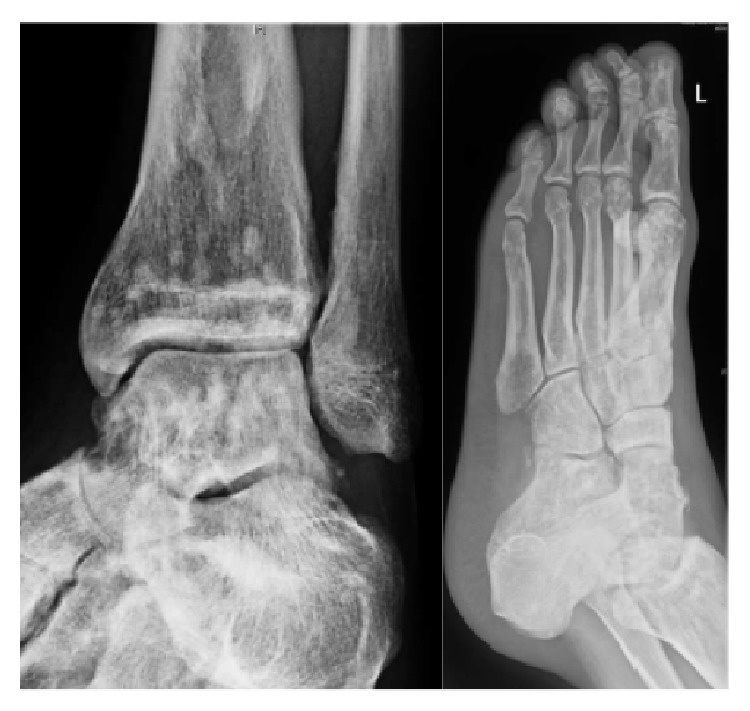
Radiograph of the left ankle and of the left foot showing multiple sclerotic spread lesions, observed at the lower part of the tibia and fibula, of the tarsal bones, metatarsal heads and predominant at the epiphyses of the phalanges.
